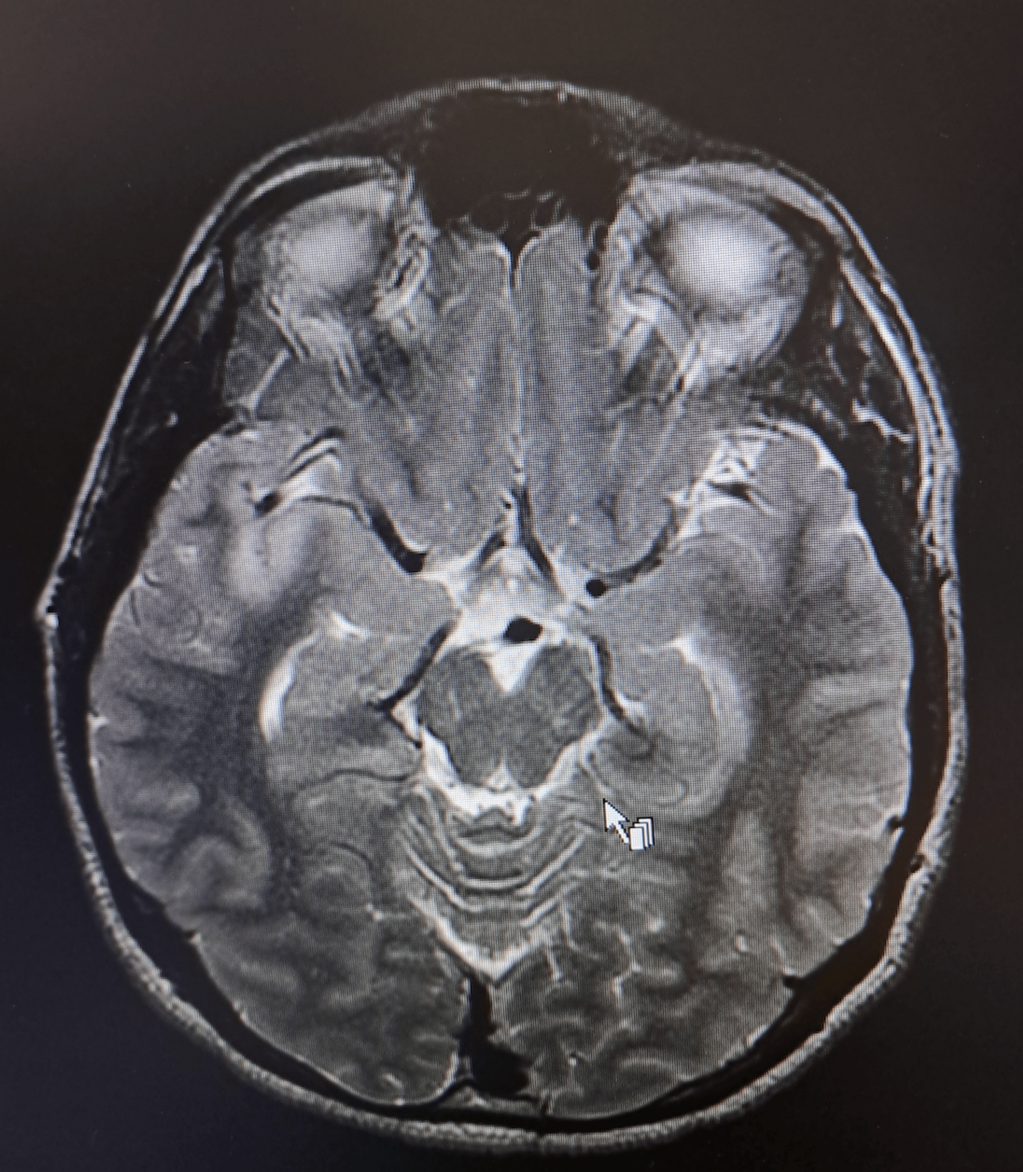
Axial T2-weighted MRI of the brain upon admission. Presence of hyperintense signal with involvement of the right temporal lobe and meningeal enhancement.
